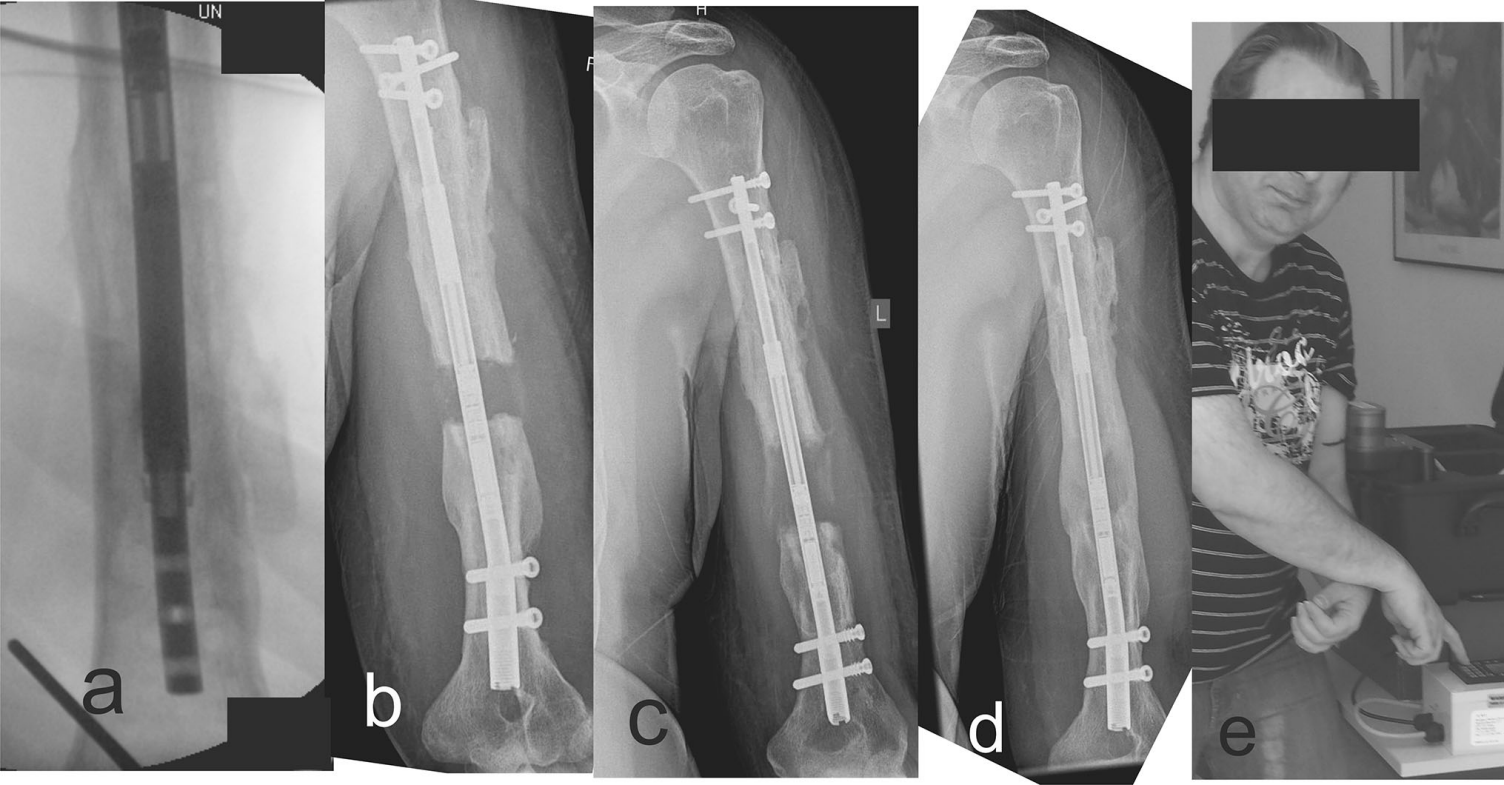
a Intraoperative radiograph of crown breakage of patient G.K.; b–d radiographs during the lengthening progress with the new PRECICE P2.1; e positioning of the external remote controller for lengthening
